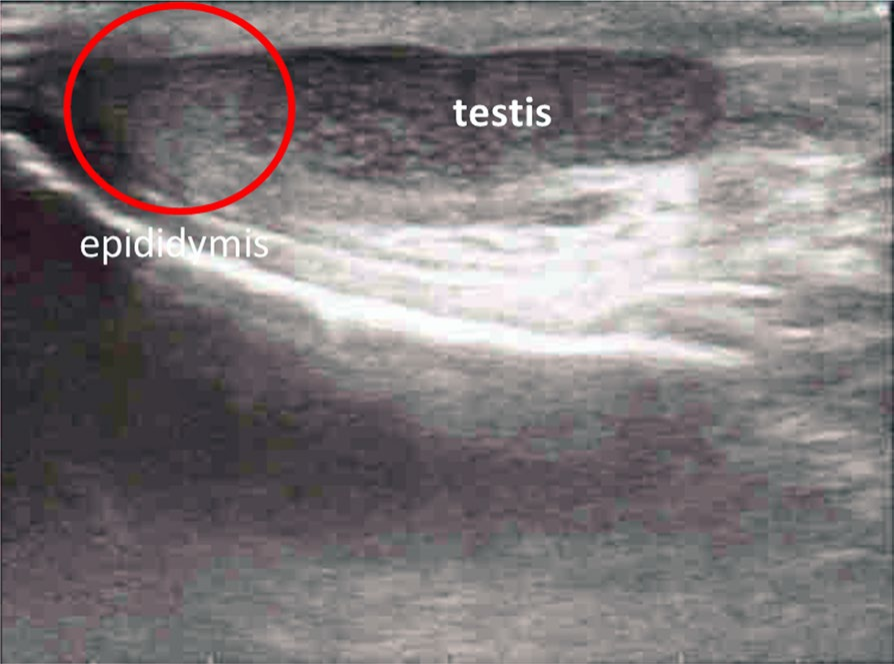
Ultrasonography of the left scrotum. No varicocele, hernia, or testicular torsion was observed. Left epididymis was swollen (red circle)
